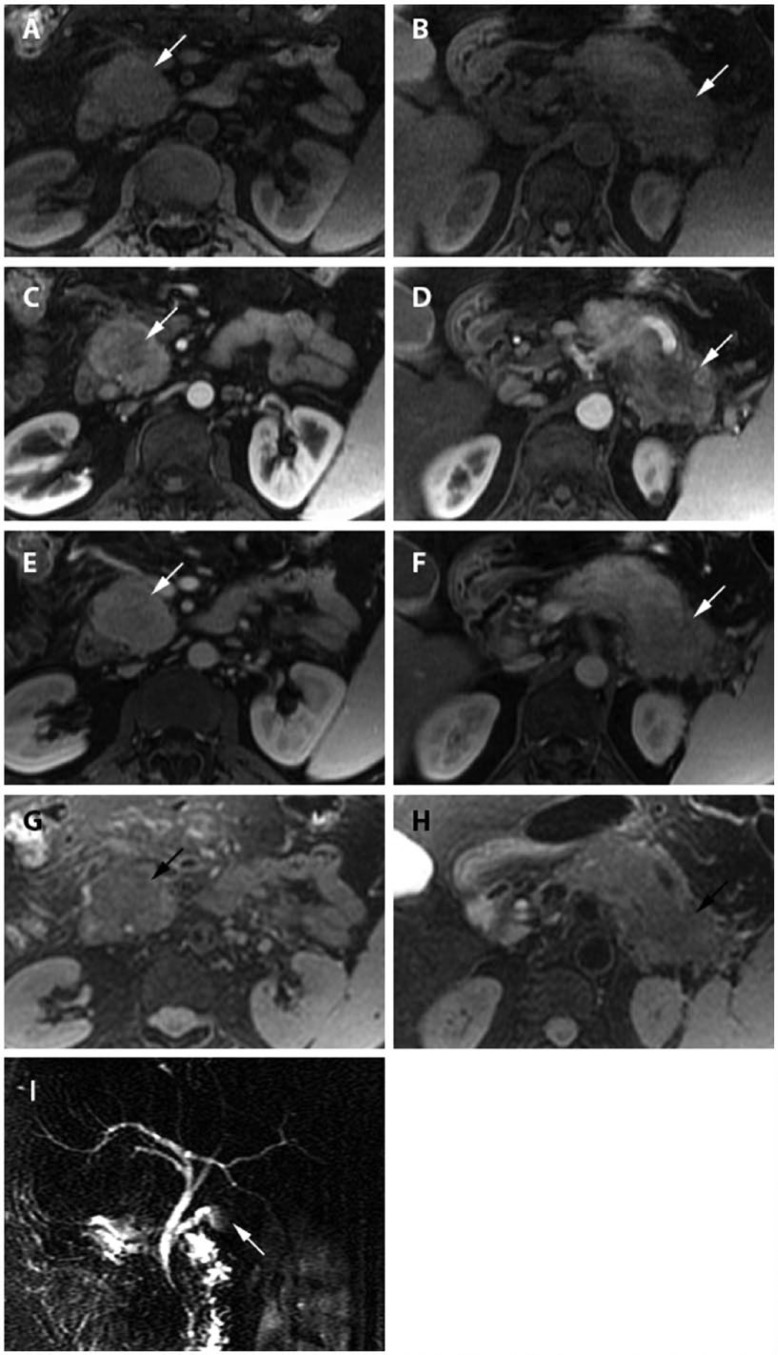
A 58-year-old female with multifocal autoimmune pancreatitis. The pancreas appears overall to be edematously swollen. (A) Axial T1-weighted MRI (LAVA) shows focal lesions in the pancreatic head (arrow) and (B) tail (arrow). (C, D) Corresponding axial contrast-enhanced T1-weighted MRI during arterial phase, (E, F) during portal venous phase, and (G, H) axial fat-saturated T2-weighted MRI during venous phase. (I) MRCP shows dilation and interruption of the pancreatic duct (arrow).
